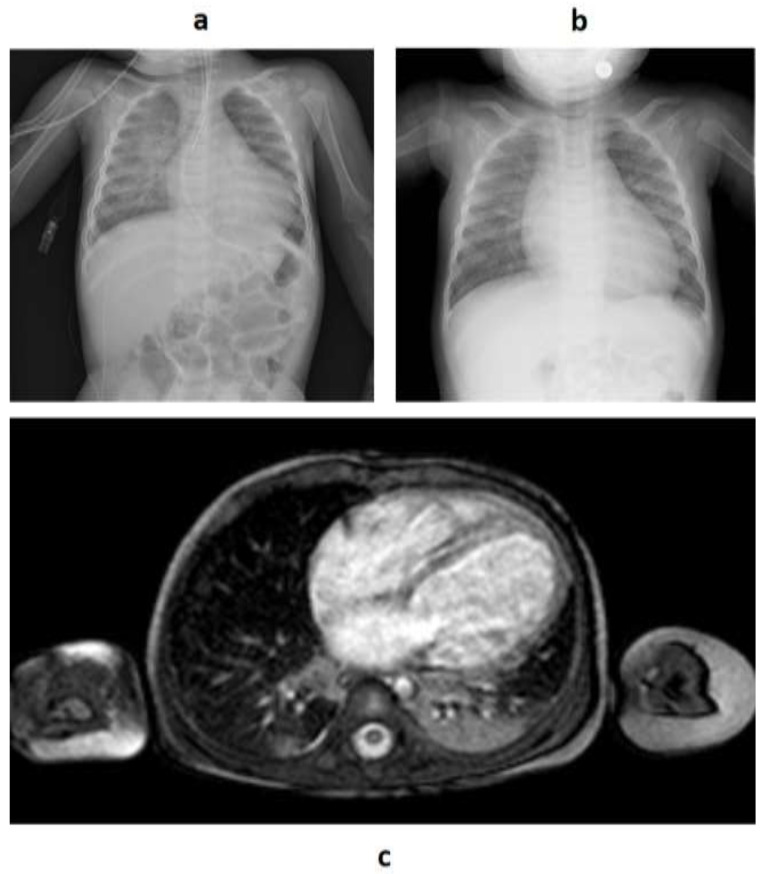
Chest X-ray imaging from patient A (a) and patient B (b) at the age of clinical onset at 7 and 6 months, respectively, showing an enlarged cardiac silhouette in both cases; (c) MRI transverse cardiac section of patient A, showing a markedly dilated left ventricle.
